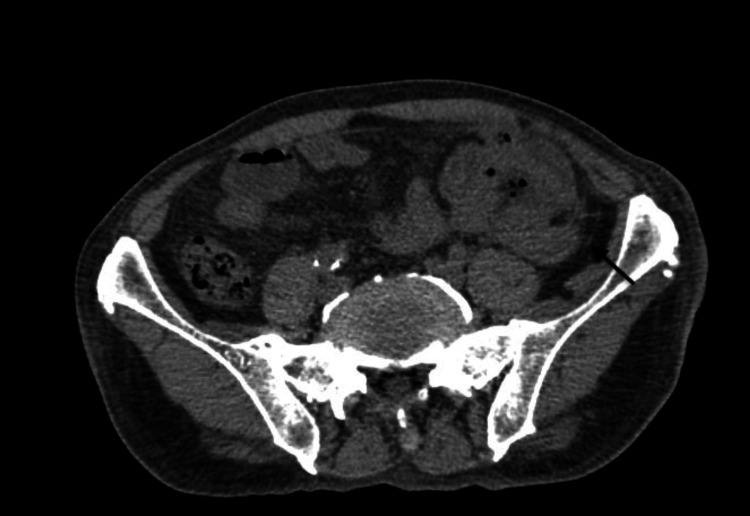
Axial CT scan showing a phlegmon in the left iliac fossa with associated pericolic fat stranding (black arrow).
